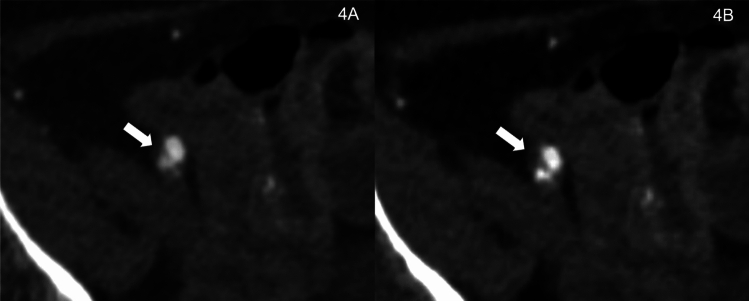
Photon-Counting CT angiography of a 68-year-old Male with diabetes and hypertension Pre- Transcatheter Aortic Valve Implantation (TAVI): Displayed are polychromatic T3D (A) and monoenergetic 55 keV reconstructions (B) of the right common femoral artery (CFA). This patient, with no history of vascular interventions, underwent PCCTA using 75 ml of 320 mg/mL iodixanol (Visipaque, GE Healthcare) at 3.5 ml/second. The T3D reconstruction of the right CFA showed a modest decrease in intraluminal iodine concentration. In contrast, the low-keV monoenergetic reconstruction at 55 keV highlighted the true vessel lumen and an intimal dissection membrane – information crucial for assessing transfemoral access and TAVI-related parameters
